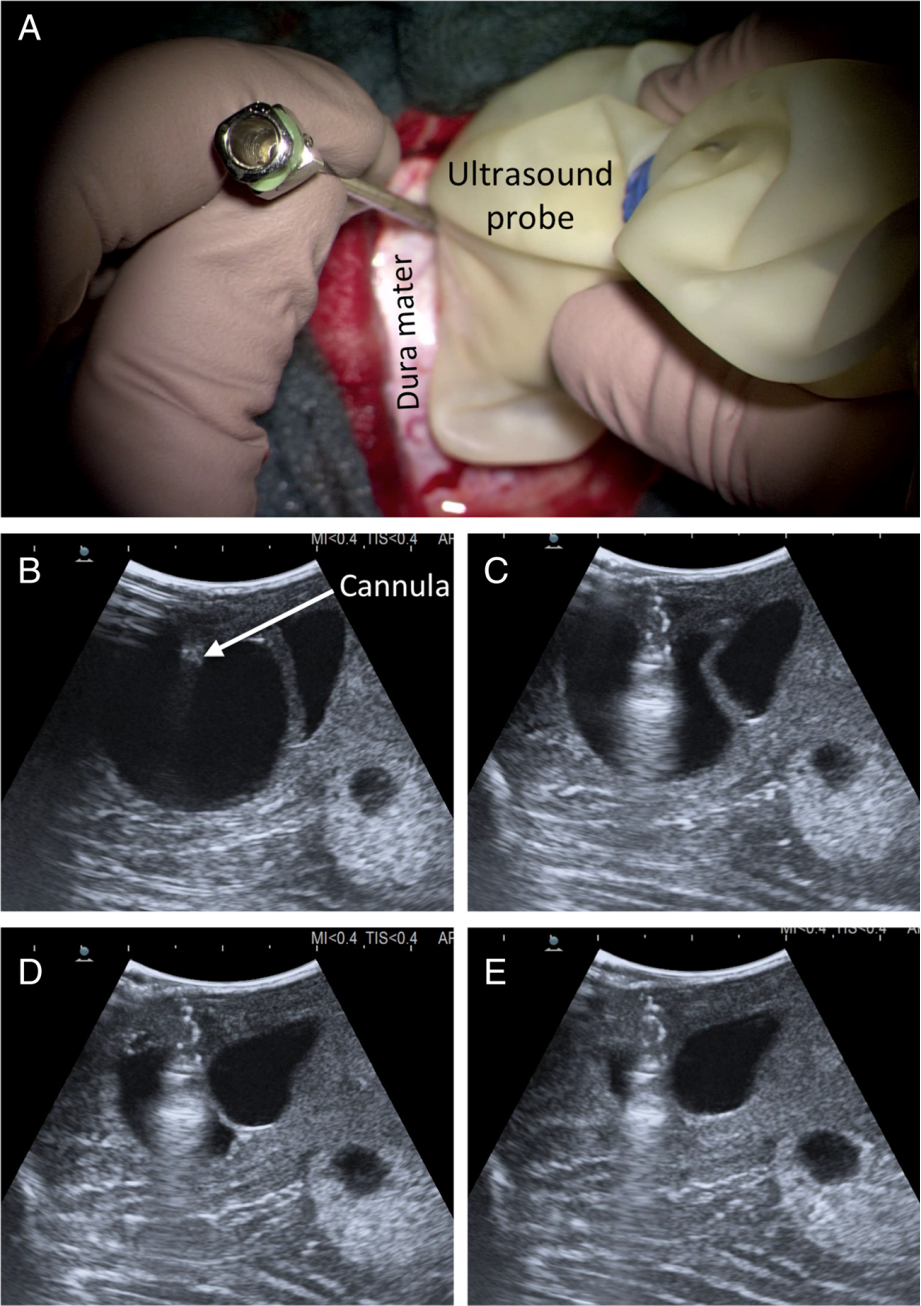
Intraoperative ultrasound-guided cyst evacuation. Intraoperatively, it may be advantageous to first evacuate a large tumor-associated cyst in order to reduce the intraparenchymal pressure. Therefore, after minimal incision of the dura, a cannula is advanced into the cyst under ultrasound guidance (a) with sequential outflow of the cyst fluid (b–e)
